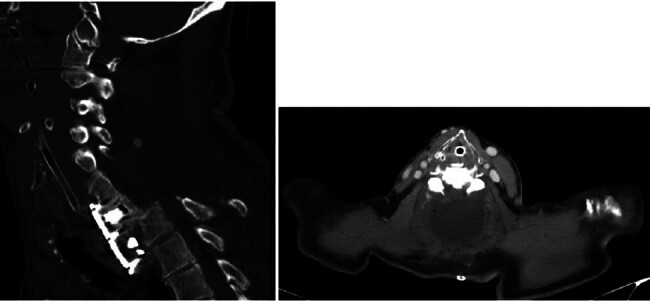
CT scan of the neck demonstrated a displaced C7–T1 segment with cerebrospinal fluid (CSF) collection around the site.
